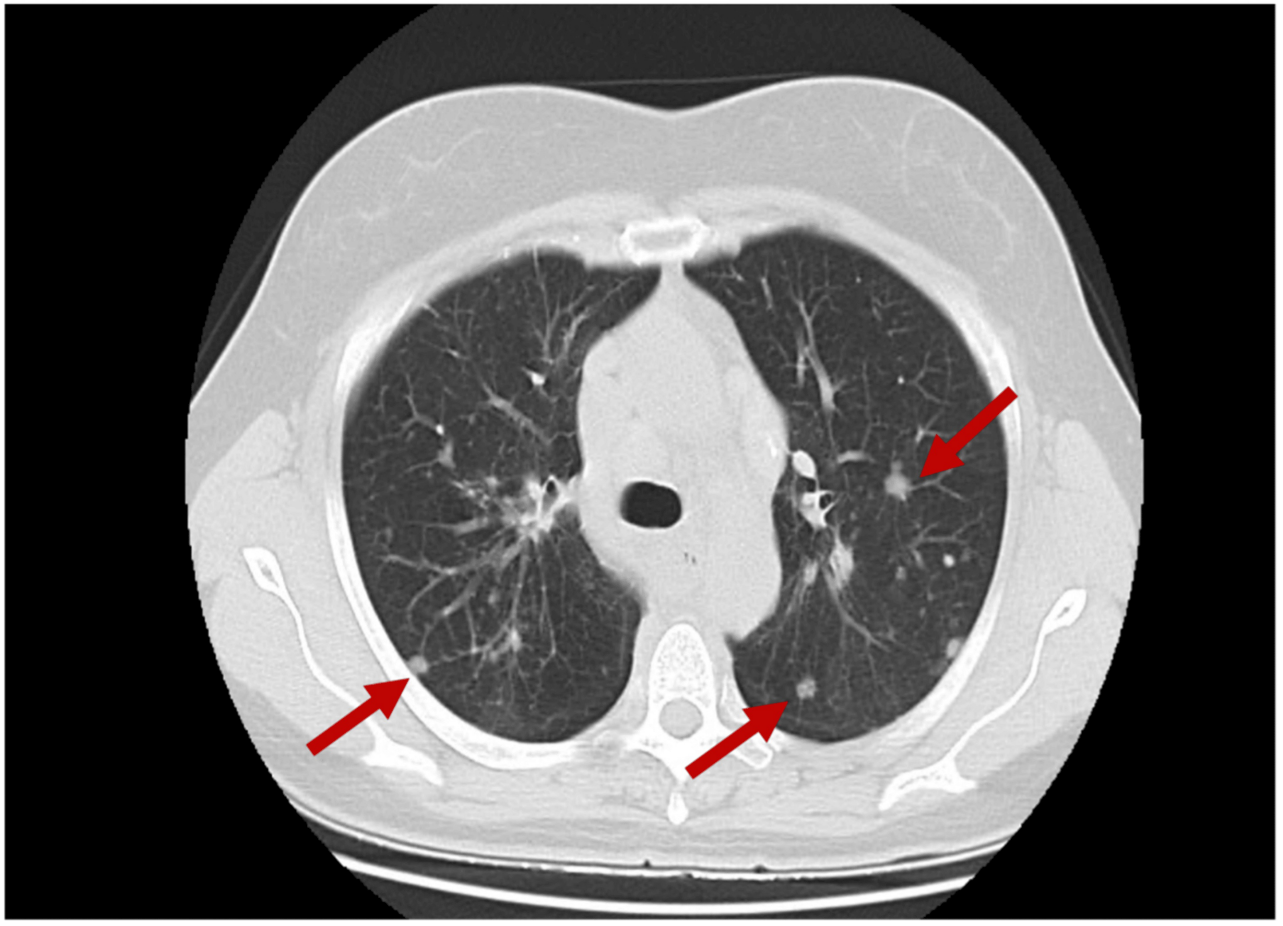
Chest CT (axial view) showing multiple solid and subsolid pulmonary nodules (red arrows).
